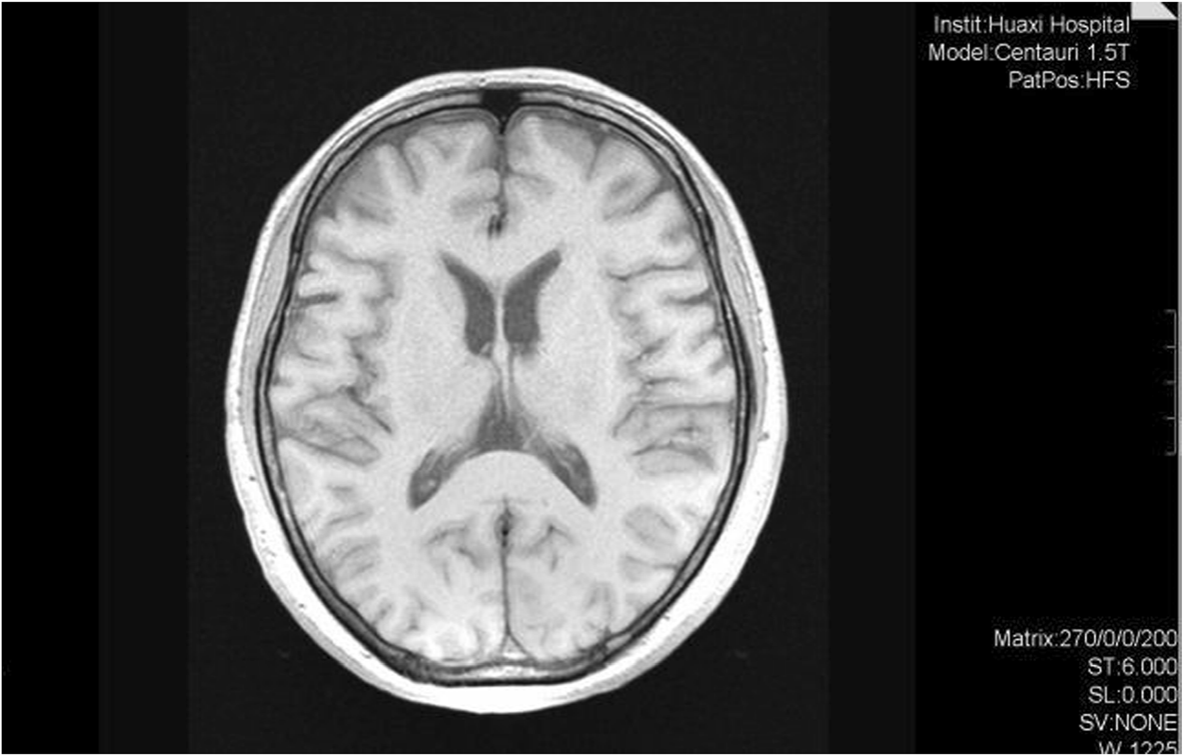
Brain MRI in 2016. No structural abnormality has been found
